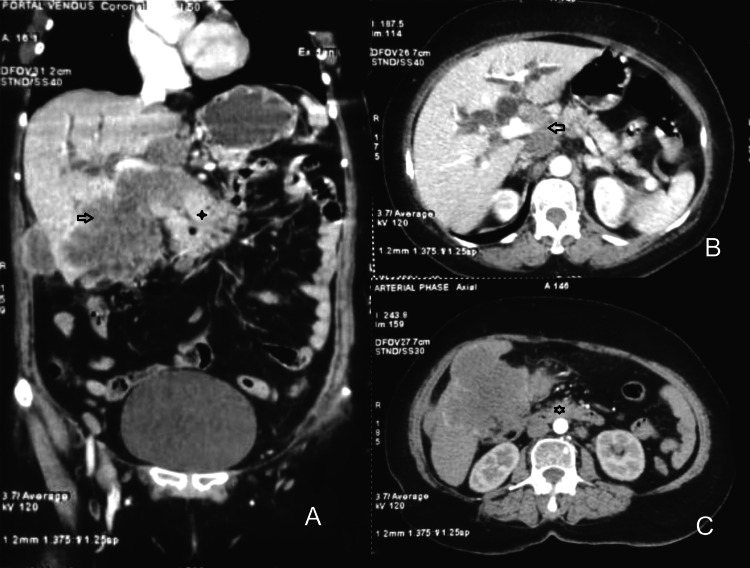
CT scan panel showing Ill-defined heterogeneous, peripherally enhancing mass lesion, with internal non-enhancing necrotic areas having exophytic component, arising from gall bladder involving segment IVb & V of the liver (arrow), medially involving antropyloric region of the stomach (plus marker), and inferiorly infiltrating hepatic flexure of the colon, also infiltrating overlying muscular layer of the anterior abdominal wall (A). The lesion is encasing the portal vein causing irregular luminal narrowing along with IHBRD due to infiltration of the common bile duct (B). Enlarged retroperitoneal lymph node noted (star marker) (C). IHBRD: intrahepatic biliary radicle dilatation
